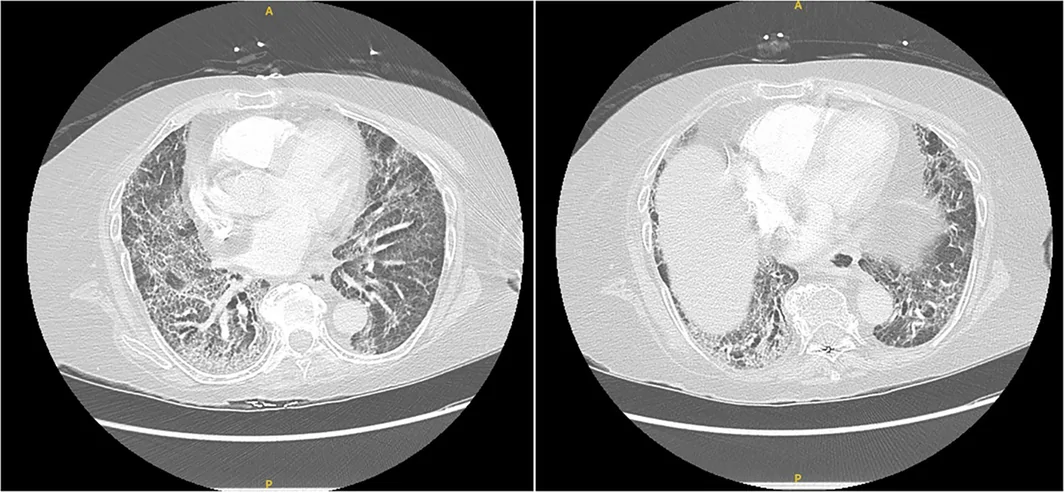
Axial HRCT of the chest at presentation. This Figure shows diffuse bilateral interstitial haziness predominantly in the mid and lower lung fields. Interlobular septal thickening is present without focal consolidation, honeycombing or pleural effusion.
